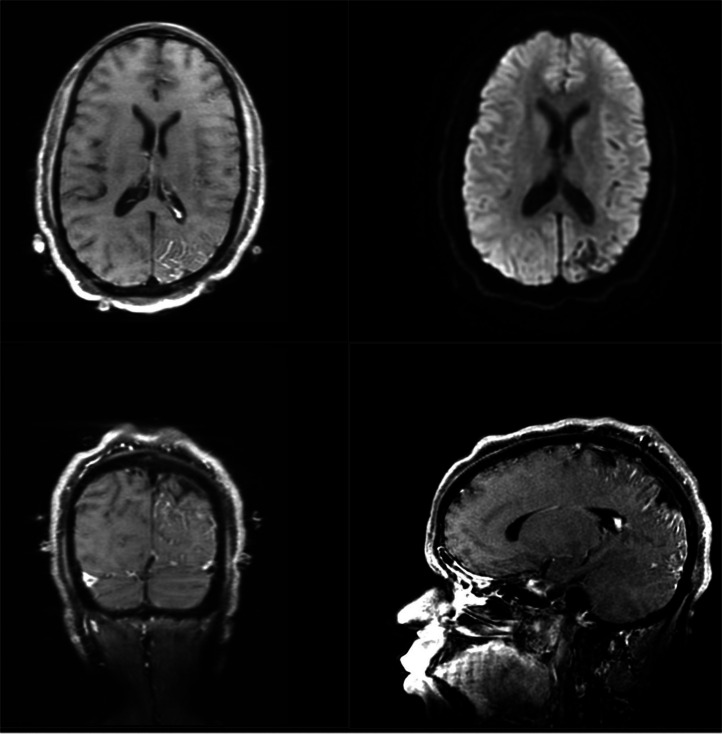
Axial DWI sequence (top right) and Axial Coronal and Sagittal T1 weighted Post contrast reformatted images showing tram-track gyriform calcification in the left parietal lobe with associated atrophy and intense gadolinium enhancement in the leptomeninges consistent with Sturge-Weber syndrome.
